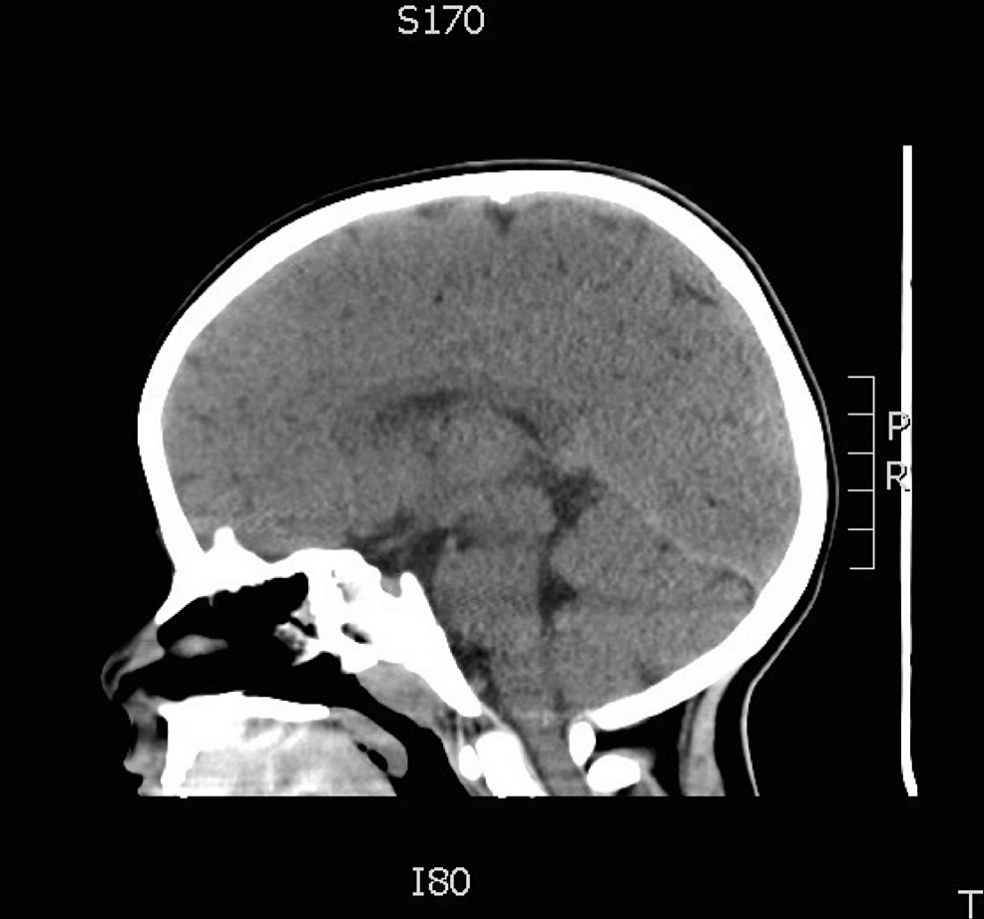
Sagittal CT performed at four years of age showing no obvious lesions in brain parenchyma.
